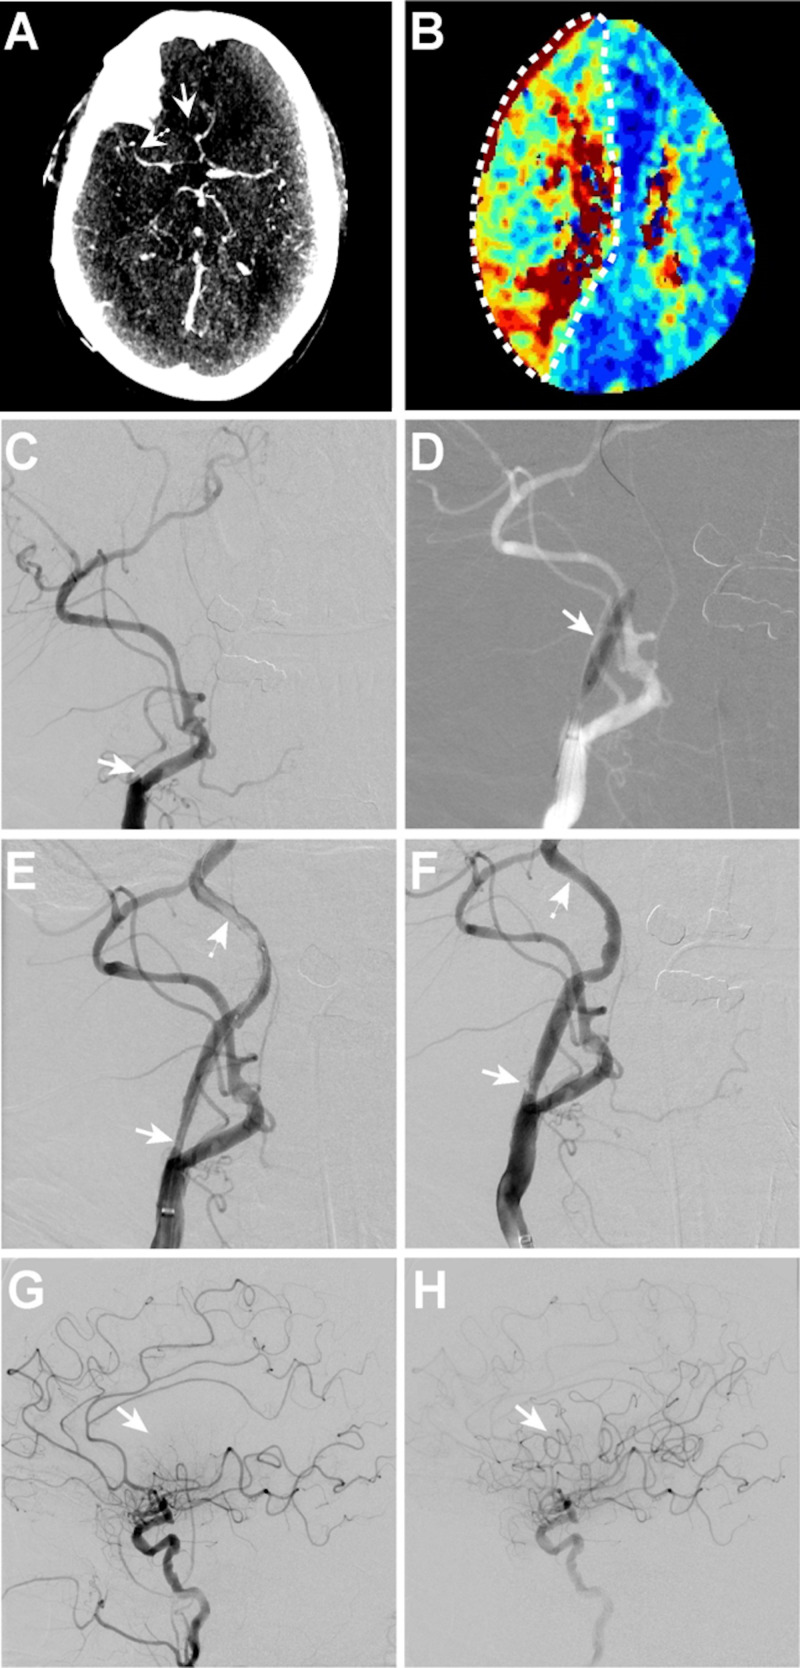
Patient 2 with right CSO eight hours post stent placement with tandem anterior cerebral artery and middle cerebral artery occlusions (A) Due to technical limitations, a CTA was not obtained, thus CT perfusion source data was reformatted into a 5mm MIP from the peak vascular enhancement series using manual bone masking at the skull base to demonstrate the pre-intervention tandem occlusions of the right A2 (arrow) and M2 (dashed arrow) segments of the anterior and middle cerebral arteries. (B) CT perfusion imaging shows a perfusion deficit (Tmax >6 seconds) in the right middle and anterior cerebral artery territory (dashed outline) secondary to the carotid stent thrombus. (C-H) Right common carotid artery DSA images. (C) There is occlusion of the right carotid stent (arrow) with no antegrade filling of the cervical right ICA (arrow). (D) Balloon angioplasty of the right carotid stent (arrow) was performed. (E) After aspiration thrombectomy and angioplasty, there is residual thrombus in the carotid stent (arrow) with additional non-occlusive thrombus in the more distal right ICA (dashed arrow). (F) After additional aspiration thrombectomy, there is minimal residual thrombus in the carotid stent (arrow) and no residual thrombus in the more distal right ICA (dashed arrow). (G) Following CSO treatment, there is poor filling of the right middle cerebral artery inferior divisions (arrow), as well as the A2 segment of the right anterior cerebral artery. (H) After combined cerebral aspiration and mechanical thrombectomy, there is excellent reperfusion of the right anterior circulation (arrow). CT, computed tomography; CTA, computed tomography angiography; ICA, internal carotid artery; DSA, digital subtraction angiography; CSO, carotid stent occlusion
